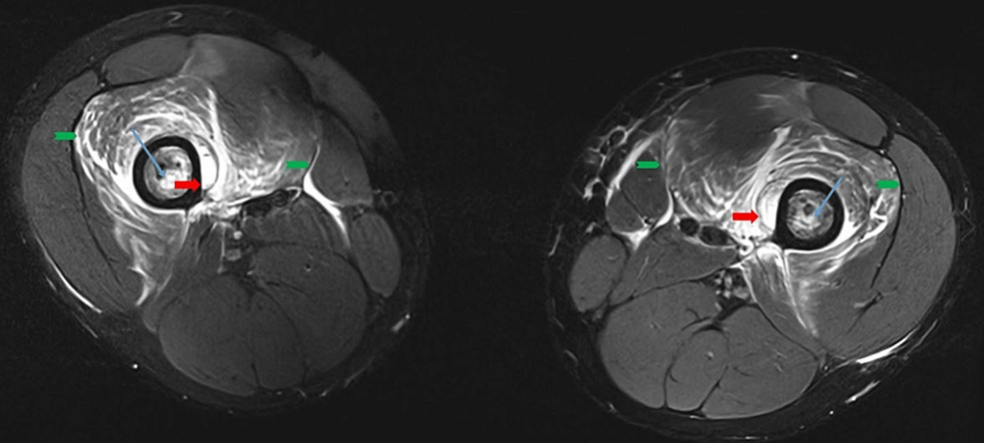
Day 6 axial MRI scan An axial T1 turbo spin echo (TSE) MRI image was taken of the lower limbs on Day 6 after hospital admission. The image on the day showed focal, high signal intensity muscle edema of the anterior compartment of the thigh, almost exclusively limited to the vastus intermedius muscle with minimal affection of the vastus medialis and biceps femoris (green arrows). Bone infarcts (blue arrow) were seen in both femur diaphysis. In addition, there was significant periosteal lifting with associated fluid signal (red arrows) medially along both femur shafts where the infarcts had occurred in the thighs.
